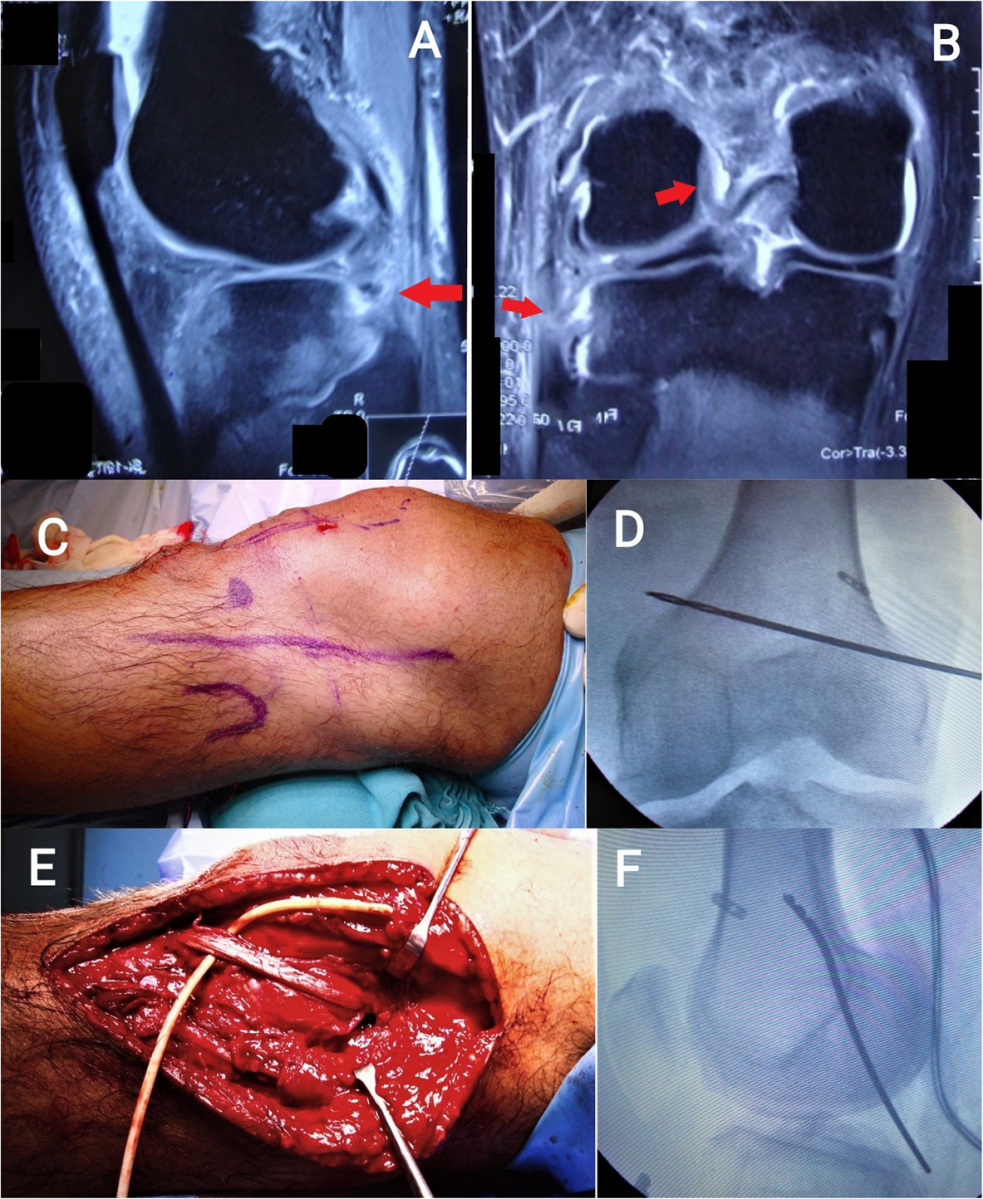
Fat-suppressed sagittal (a) and coronal (b) MRI images of a patient showing ACL and PLC injury tears (*red arrows* show discontinuity of ACL fibres from the femoral attachments and discontinuity of LCL and popliteus tendon). c Skin incision marked for reconstruction of PLC. d, f Intraoperative fluoroscopic images showing position of Beath pin in anteroposterior and lateral views. e Intraoperative clinical image showing semitendinosus tendon graft secured in the femur and the fibular head
